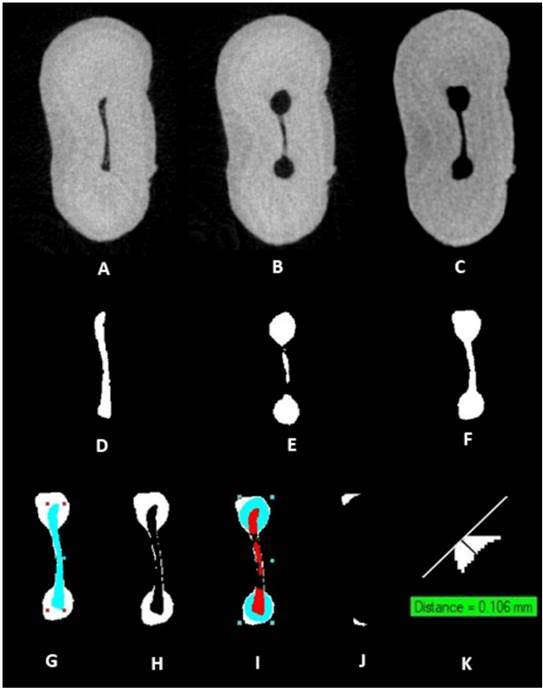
Microtomographic sections of the apical third of a sample, demonstrating Uncontrolled Dentin Removal analysis. (A) Cross-sectional image showing the preoperative root canal. (B) Cross-sectional image showing the prepared canal. (C) Cross-sectional image showing the canal after ultrasonic activation. (D) Segmented preoperative canal. (E) Segmented prepared canal. (F) Segmented root canal after ultrasonic activation. (G, H, and I) Superimposition and subtraction of D, E, and F; (J) Segmented Uncontrolled Removed Dentin. (K) Measurement of maximum depth of defects.
